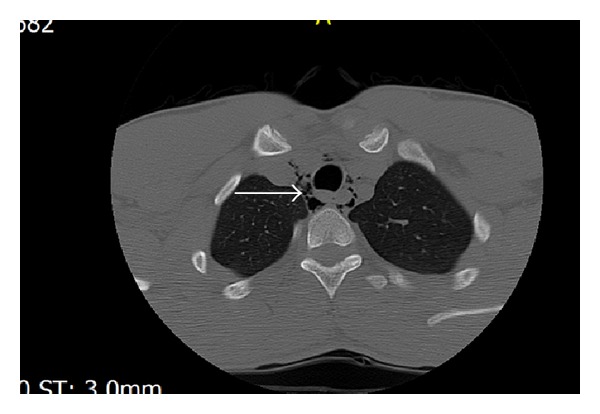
Mediastinal CT scan, axial section; free air is present around trachea and in mediastinum (arrow).
